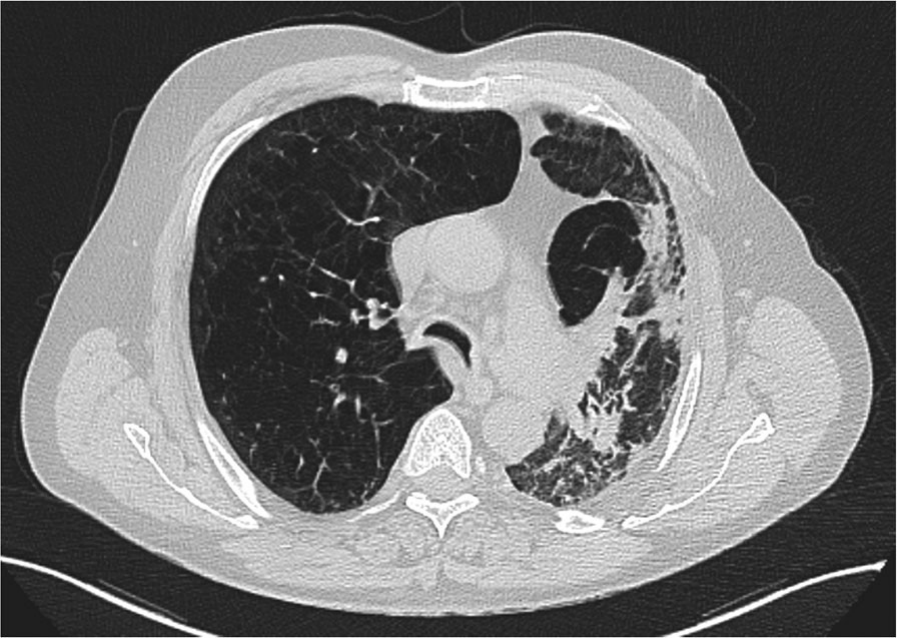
Chest computed tomography performed on day 1 showing diffused centrilobular emphysema and a fibrothorax aspect. Air bronchograms were evidenced bilaterally at bases
